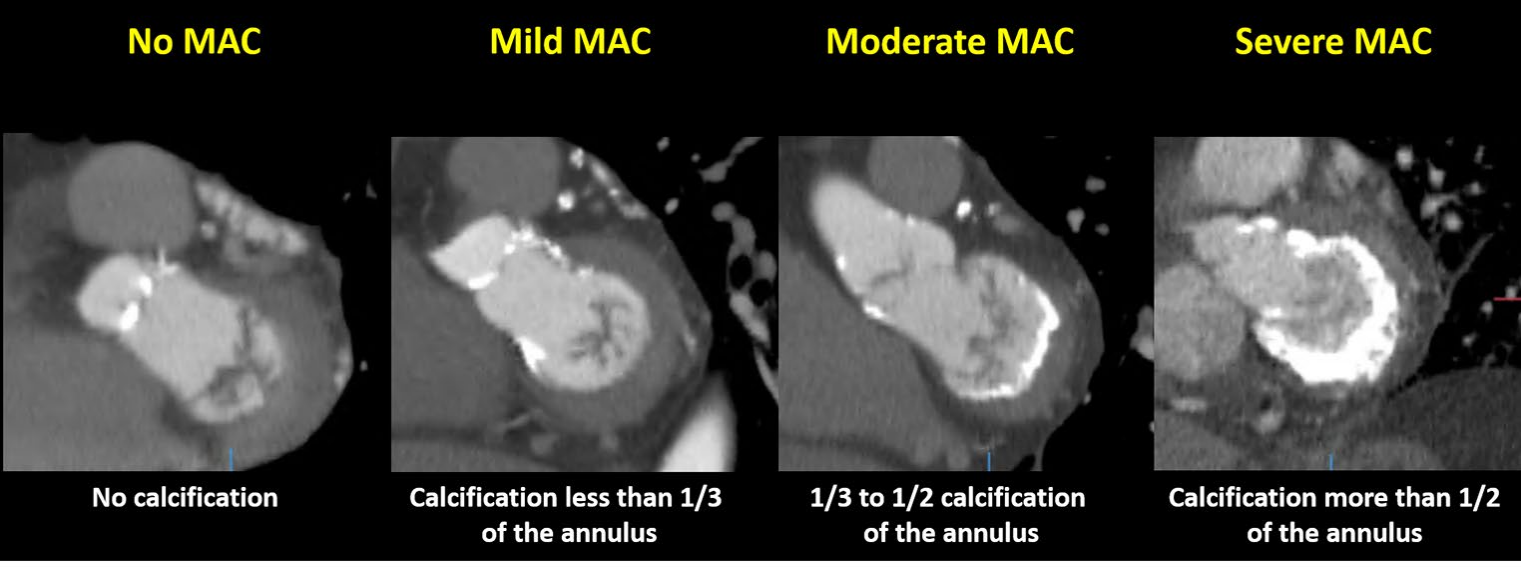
CT Evaluation of MAC. MAC severity was quantitively determined according to the circumferential involvement of calcification in mitral annulus MAC = mitral annular calcification
